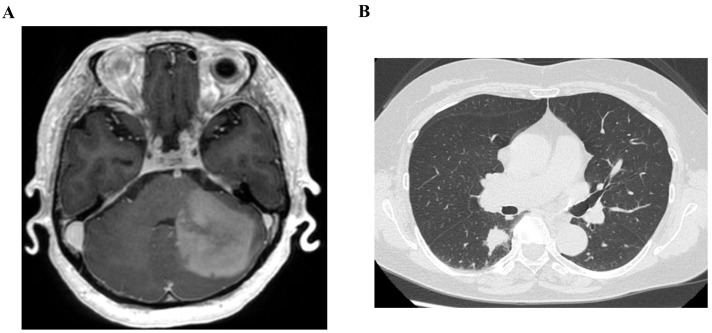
Radiologic features of synchronous glioblastoma and lung adenocarcinoma (A) Contrast-enhanced magnetic resonance imaging of the brain reveals a tumor lesion extending from the left cerebellar hemisphere to the medulla oblongata. (B) Chest computed tomography showing a spiculated nodule in the right lower lobe of the lung.
